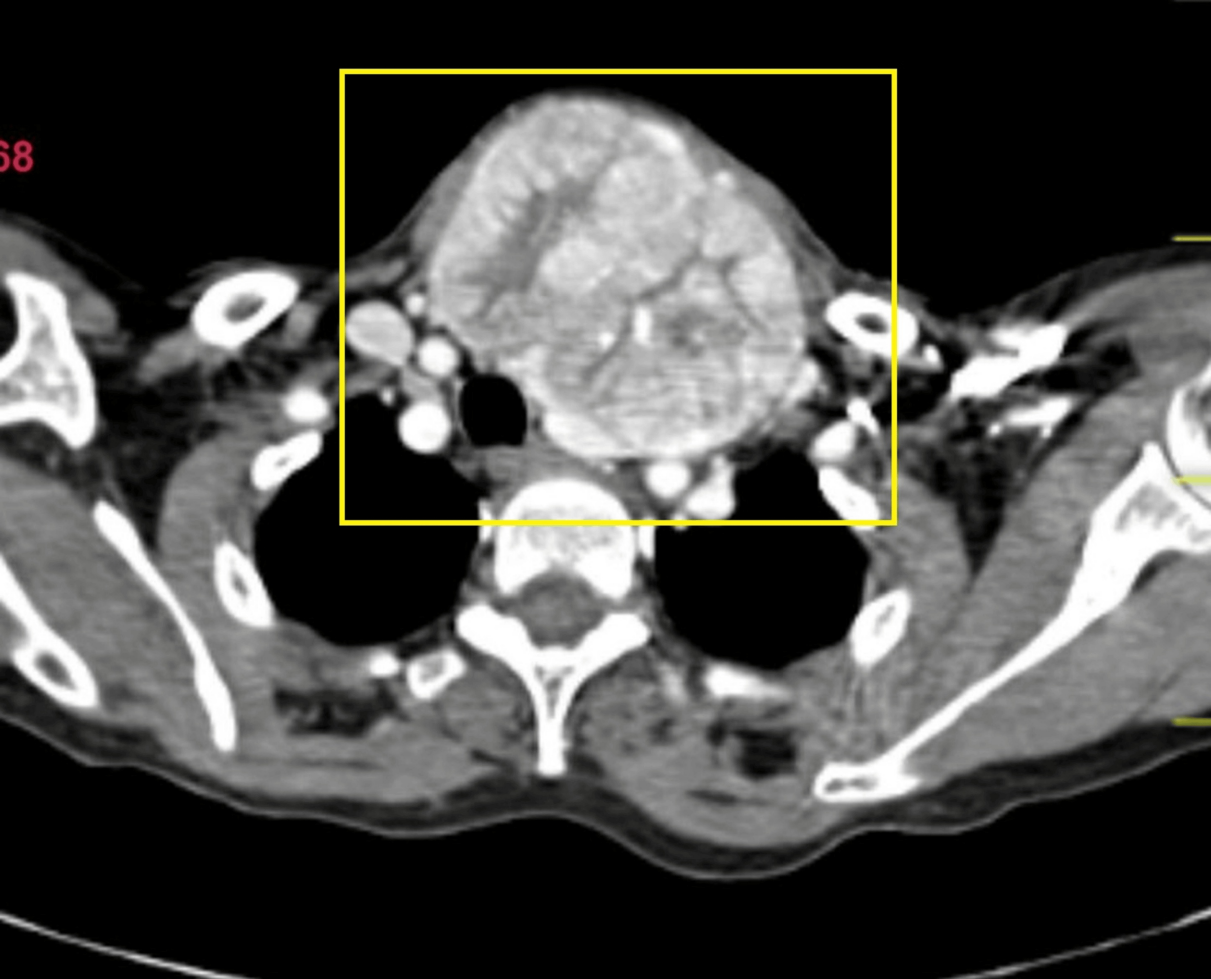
CECT showing thyroid nodule axial view showing heterogeneously enhanced soft tissue lesion with mass effect over trachea causing deviation of trachea towards the right side. CECT: contrast-enhanced computed tomography
